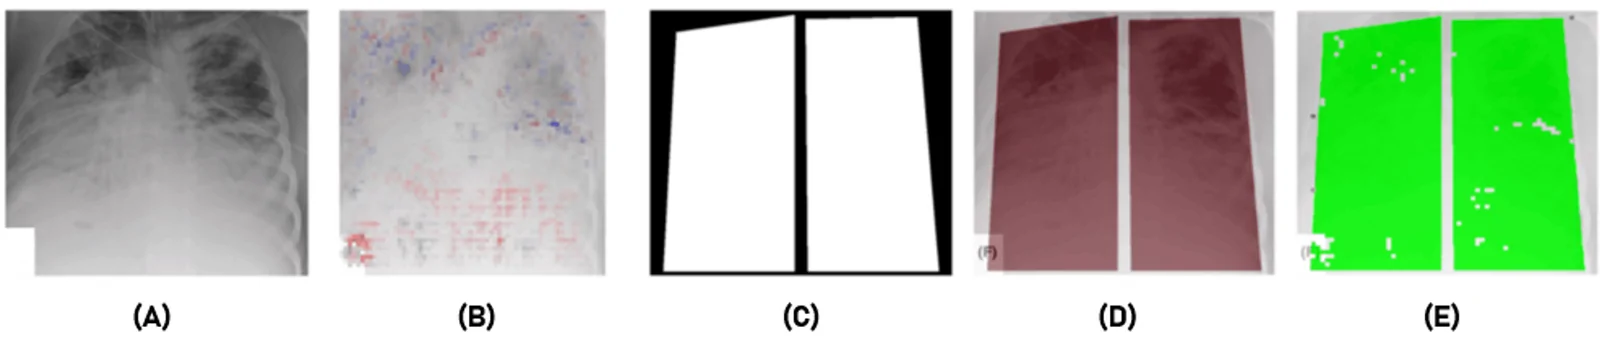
(A) COVID-19 without pneumonia chest X-ray; (B) LRP relevance map (α = 2, β = 1); (C) binary lung mask; (D) lung mask overlaid on the X-ray (region of interest); (E) IoU overlay highlighting overlap between thresholded relevance and lung regions, with the IoU value shown. COVID-19: coronavirus disease 2019; LRP: layer-wise relevance propagation; IoU: intersection over union Panel (A) was adapted from the Covid_w/wo_pneumonia chest Xray dataset [19] and is used under the Community Data License Agreement-Sharing 1.0 (CDLA-Sharing-1.0); panels (B-E) and all overlays and annotations were generated by the authors.
